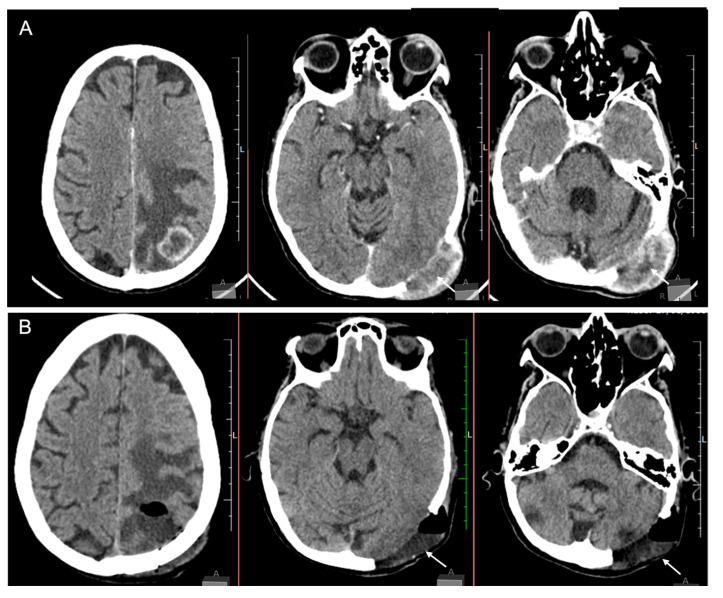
Computer tomography (CT scan) of the brain showing pre- (A) and postoperative images (B). (A) CT scan with venous contrast showing an intra-axial lesion on the occipital/parietal lobes and an infiltrative transcranial lesion in the occipital bone. (B) Postoperative image of both intracranial and transcranial lesions.
